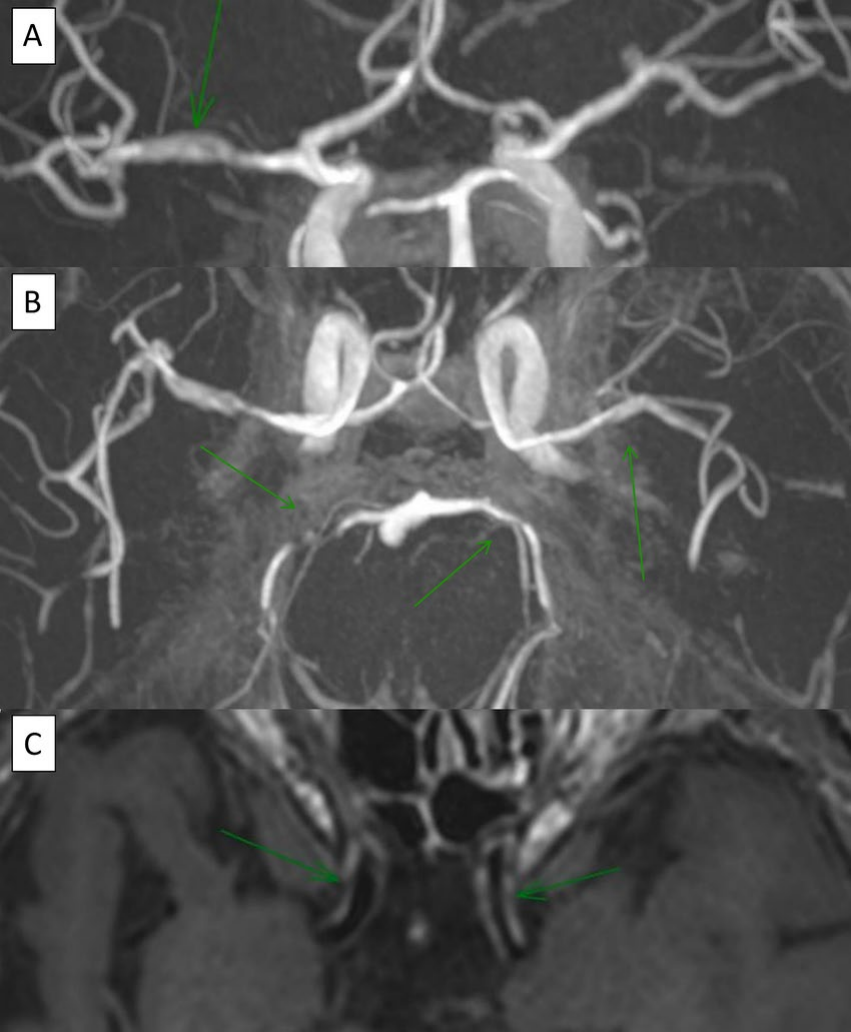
A 70-year-old patient with medical history of multiples acute ischemic stroke. Time-of-flight (TOF) brain MRI reveals multiple intracranial stenoses (A, coronal view; B, axial view), while vessel wall MRI (VWI) demonstrates circumferential contrast enhancement (C, axial view).
